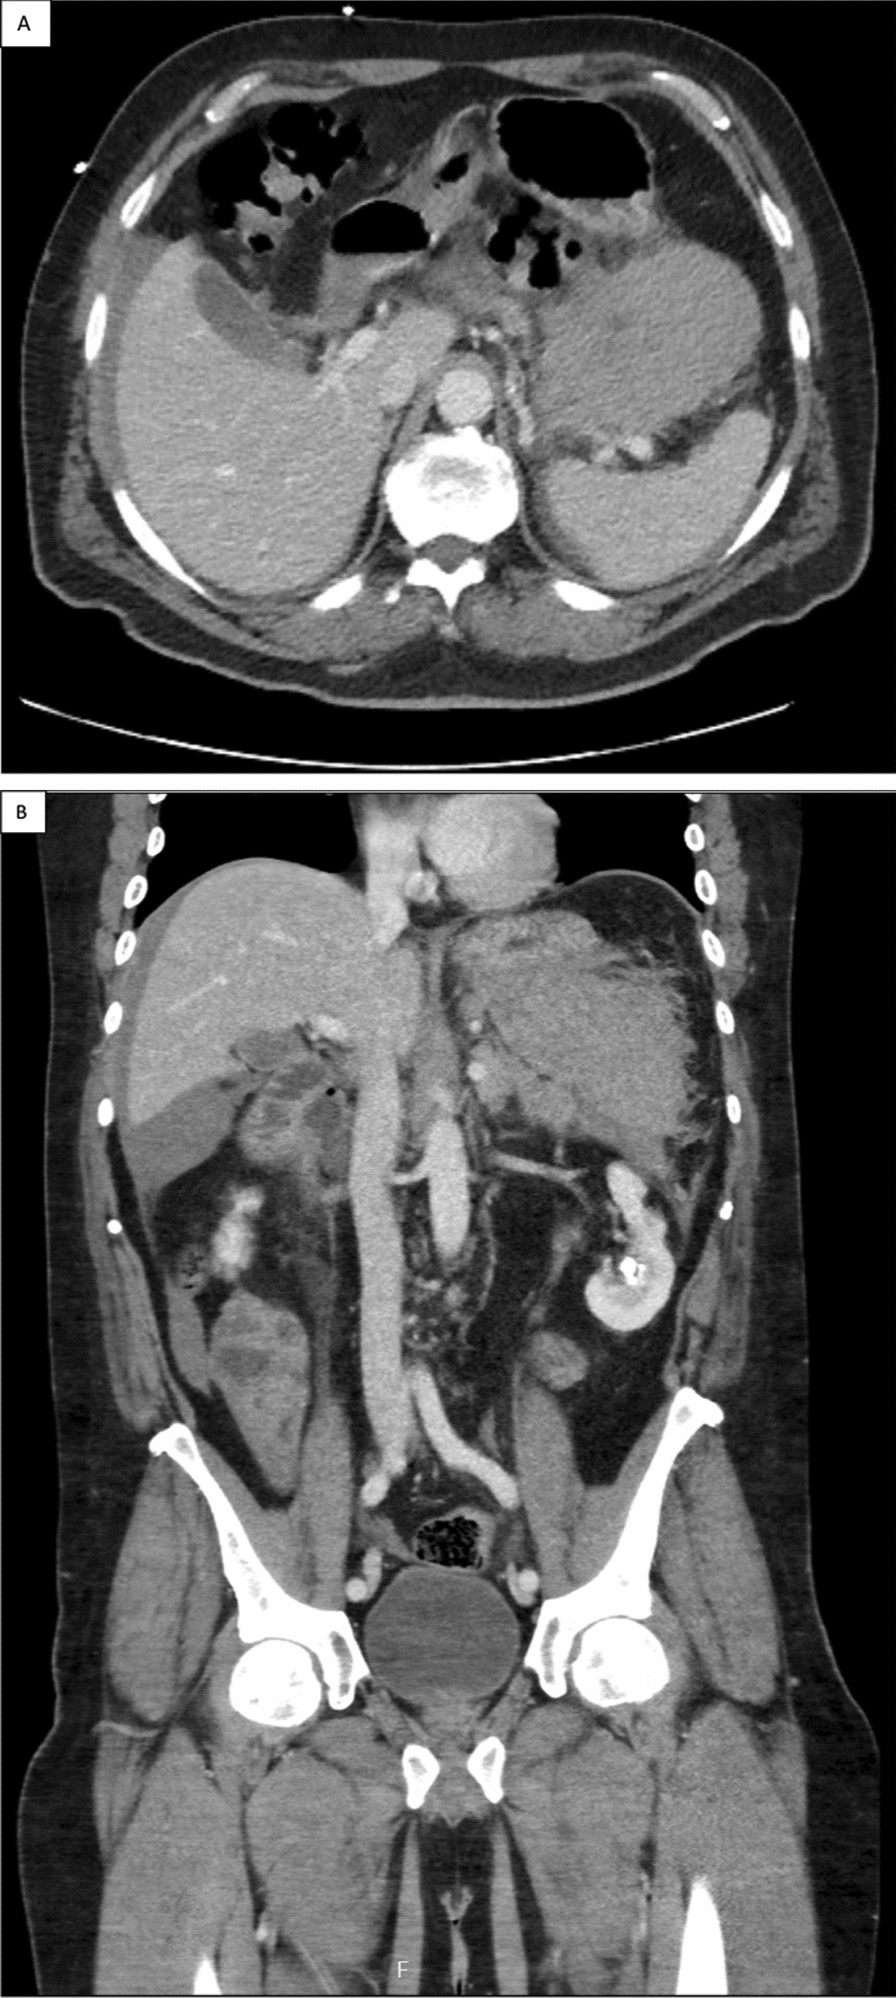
A Abdominal computed tomography revealed a 10.3 cm × 6.5 cm × 10.0 cm hematoma partially encasing the pancreatic tail, displacing the stomach anteriorly and medially. B Coronal visualization of hematoma
